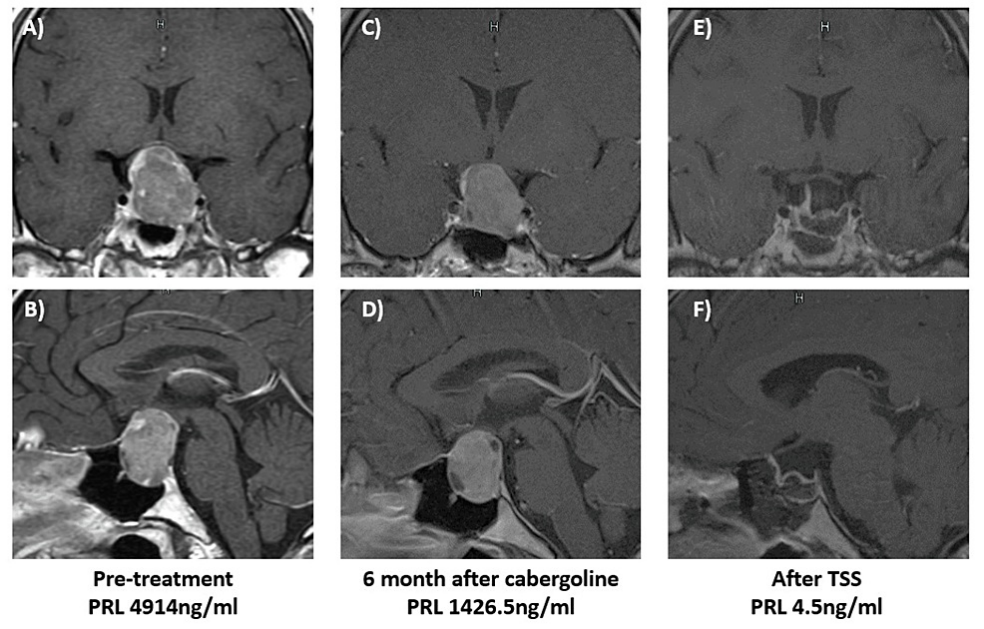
A patient who showed resistance to cabergoline therapy (Case 8) A, B: Pretreatment MRI scan. MRI scan of the head showed a tumor in the sella turcica, with a maximum diameter of 25 mm; C, D: After cabergoline treatment, PRL levels decreased, but there was no tumor shrinkage; E, F: Postsurgery. The tumor was completely removed, and the PRL has normalized. PRL: prolactin; TSS: transsphenoidal surgery
